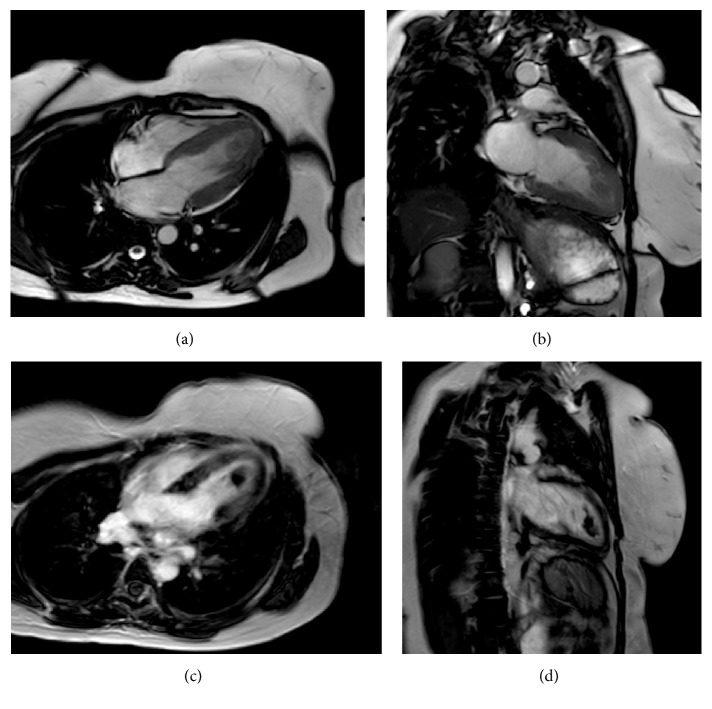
Cardiac magnetic resonance imaging showing a thrombus in the left ventricule apex and late gadolinium enhancement (LGE). (a) Four-chamber view: ventricular thrombus measuring 25 × 30 mm; (b) two-chamber view: ventricular thrombus extending to the anterior wall; (c) four-chamber view (10 min of gadolinium injection): LGE in inferoseptal and anterolateral walls and in the apex; (d) two-chamber view (10 min of gadolinium injection): LGE in the anterior wall and in the apex.
